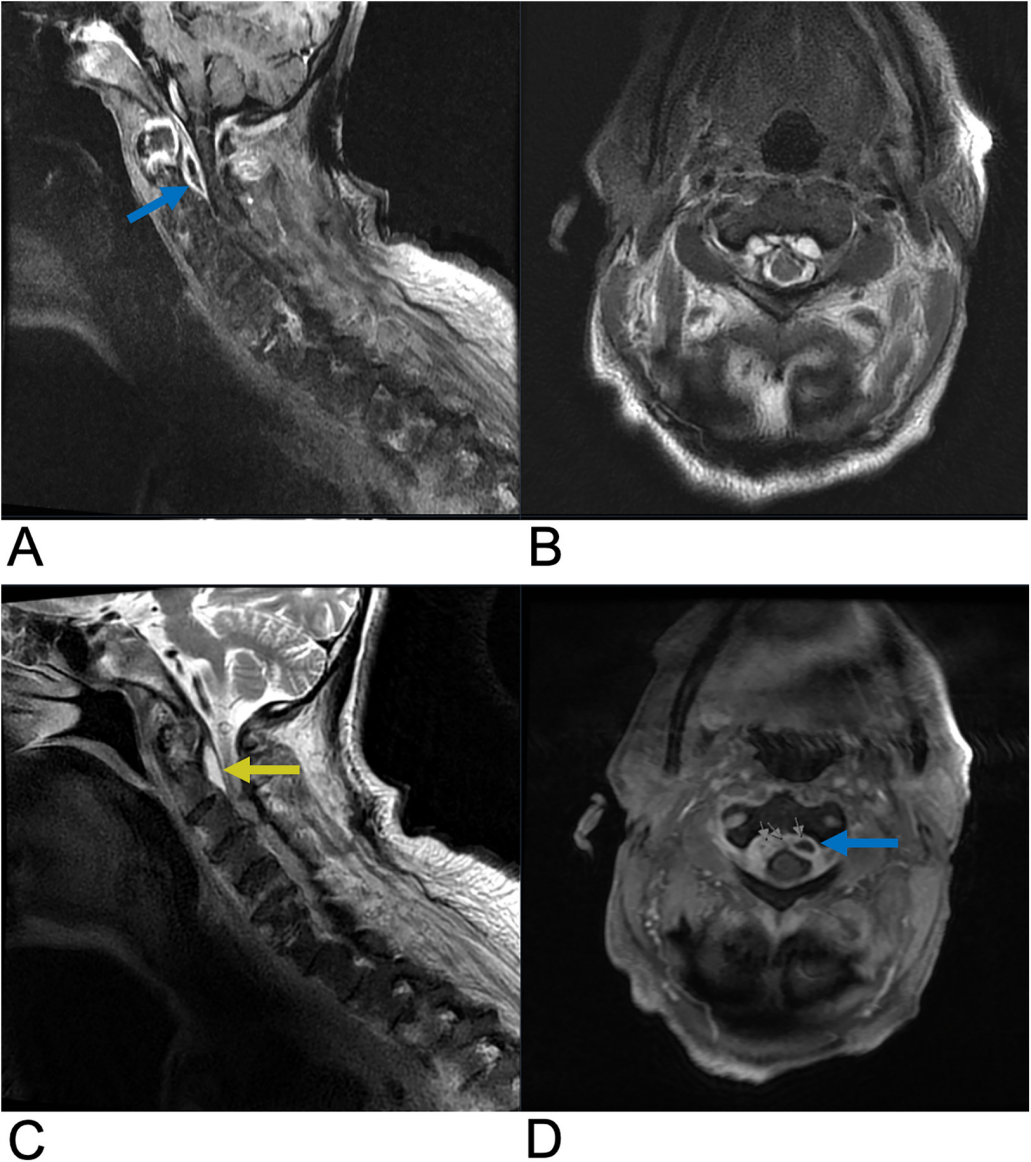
Epidural abscess. Sagittal T1 + C (A), axial T2 (B), sagittal T2 (C), and axial T1 + C (D) MRI demonstrating a T2 hyperintense lesion (yellow arrow) at the epidural space encroaching on the spinal canal and causing spinal cord compression. The abscess is peripherally enhancing on post-contrast T1 weighted MRI (blue arrows) consistent with surrounding inflammatory tissue.
